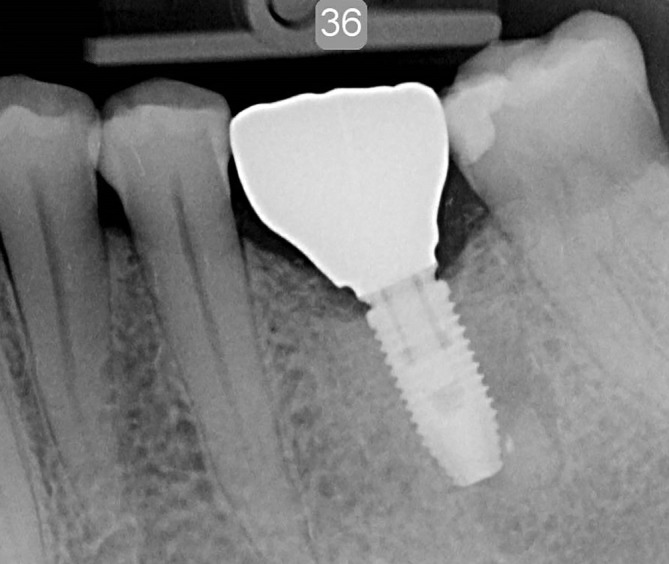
Intraoral radiograph of a parallel‐walled implant with a full‐contour zirconia restoration and angulated screw channel abutment
